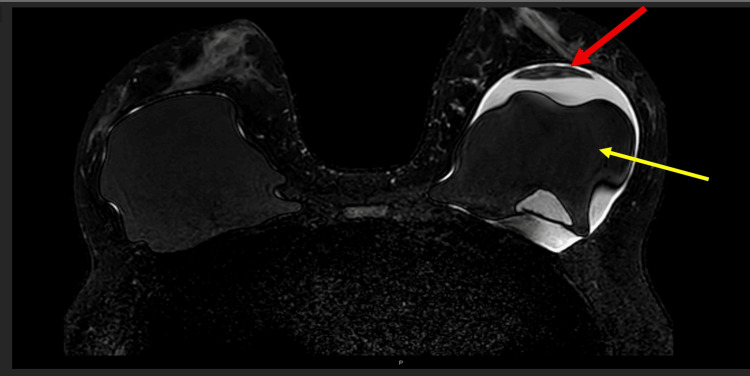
The left silicone implant (yellow arrow) and floating piece of silicone (red arrow) appeared dark in the silicone-suppressed MRI scan of the breasts. MRI: magnetic resonance imaging
